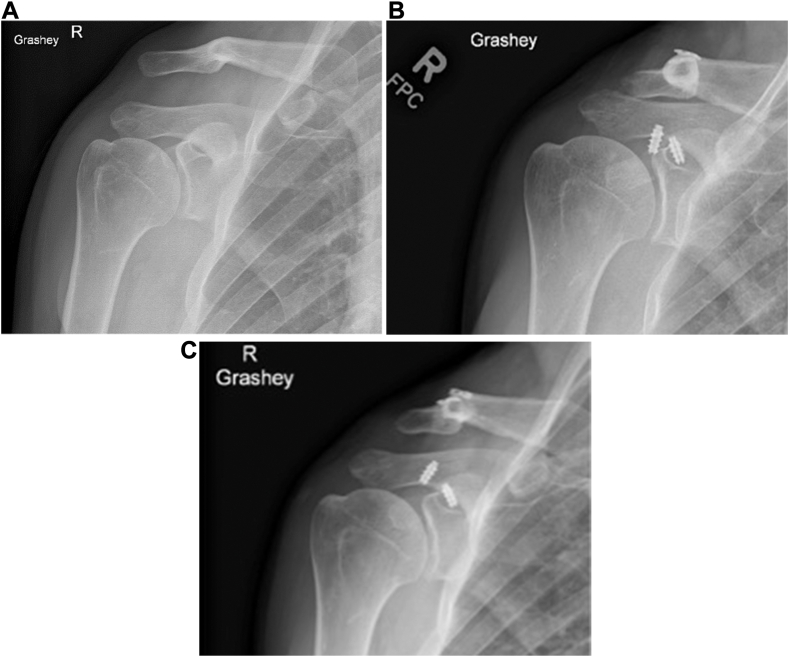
(A) Preoperative X-ray imaging (Grashey view) demonstrating Rockwood type 5 ACJ dislocation. (B) Two weeks postoperative X-ray imaging (Grashey view) demonstrating partial anchor loosening, with no loss of ACJ reduction. (C) Six weeks postoperative X-ray imaging (Grashey view) demonstrating anchor pullout and loss of ACJ reduction. *ACJ*, acromioclavicular joint.
